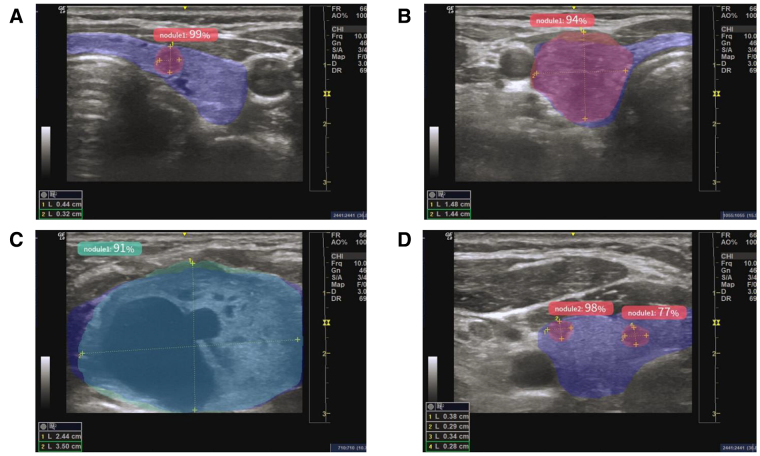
Ultrasound images of TNs. (A) A patient with papillary thyroid carcinoma (PTC) (female, 38 years). The nodule has a maximum diameter of 4.4 mm, is located in the right lobe near the isthmus, and is situated <2 mm from the capsule, which is smooth and intact. No HT was present. The ultrasonographer classified it as ACR TI-RADS 4b, while AI-UADS indicated a malignant probability of 99%. (B) A patient with PTC (female, 47 years). The nodule has a maximum diameter of 14.8 mm, is located in the left lobe, and is situated <2 mm from the capsule, which is interrupted. No HT was present. The ultrasonographer classified it as ACR TI-RADS 5, while AI-UADS indicated a malignant probability of 94%. (C) A patient with nodular goiter (female, 62 years). The nodule has a maximum diameter of 35.0 mm, is located in the right lobe, and is situated <2 mm from the capsule, which is smooth and intact. No HT was present. The ultrasonographer classified it as ACR TI-RADS 3, while AI-UADS indicated a benign probability of 91%. (D) A patient with PTC (female, 42 years). Nodule 1 has a maximum diameter of 3.8 mm, is located in the left lobe, and is situated <2 mm from the capsule, which is interrupted. HT was present. The ultrasonographer classified it as ACR TI-RADS 5, while AI-UADS indicated a malignant probability of 98%. Nodule 2 has a maximum diameter of 3.4 mm, is located in the left lobe, and is situated <2 mm from the capsule. The ultrasonographer classified it as ACR TI-RADS 4a, while AI-UADS indicated a malignant probability of 77%. AI-UADS = artificial intelligence ultrasound-assisted diagnostic system, HT = Hashimoto thyroiditis, PTC = papillary thyroid carcinoma, TNs = thyroid nodules, TI-RADS = Thyroid Imaging Reporting and Data System.
